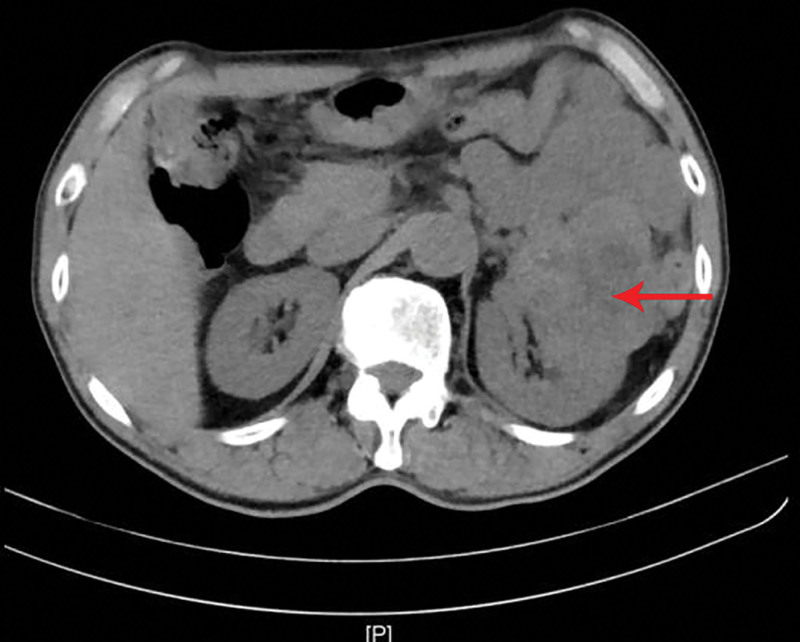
Abdominal CT demonstrates an irregular mass with ill-defined margins and heterogeneous density in the left renal region (as indicated by the arrow). CT = computed tomography.
